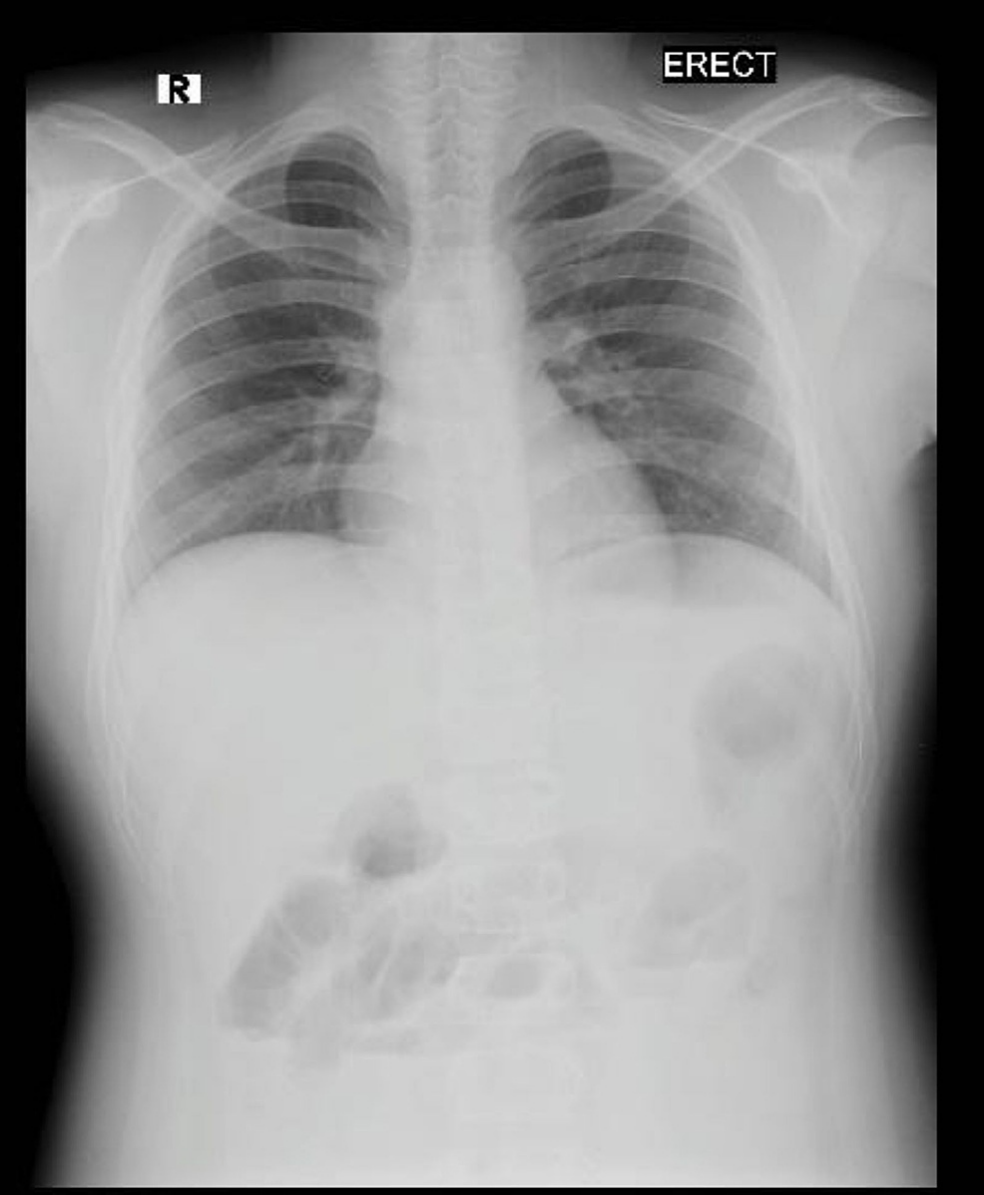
Erect chest X-ray showing normal anatomy.
